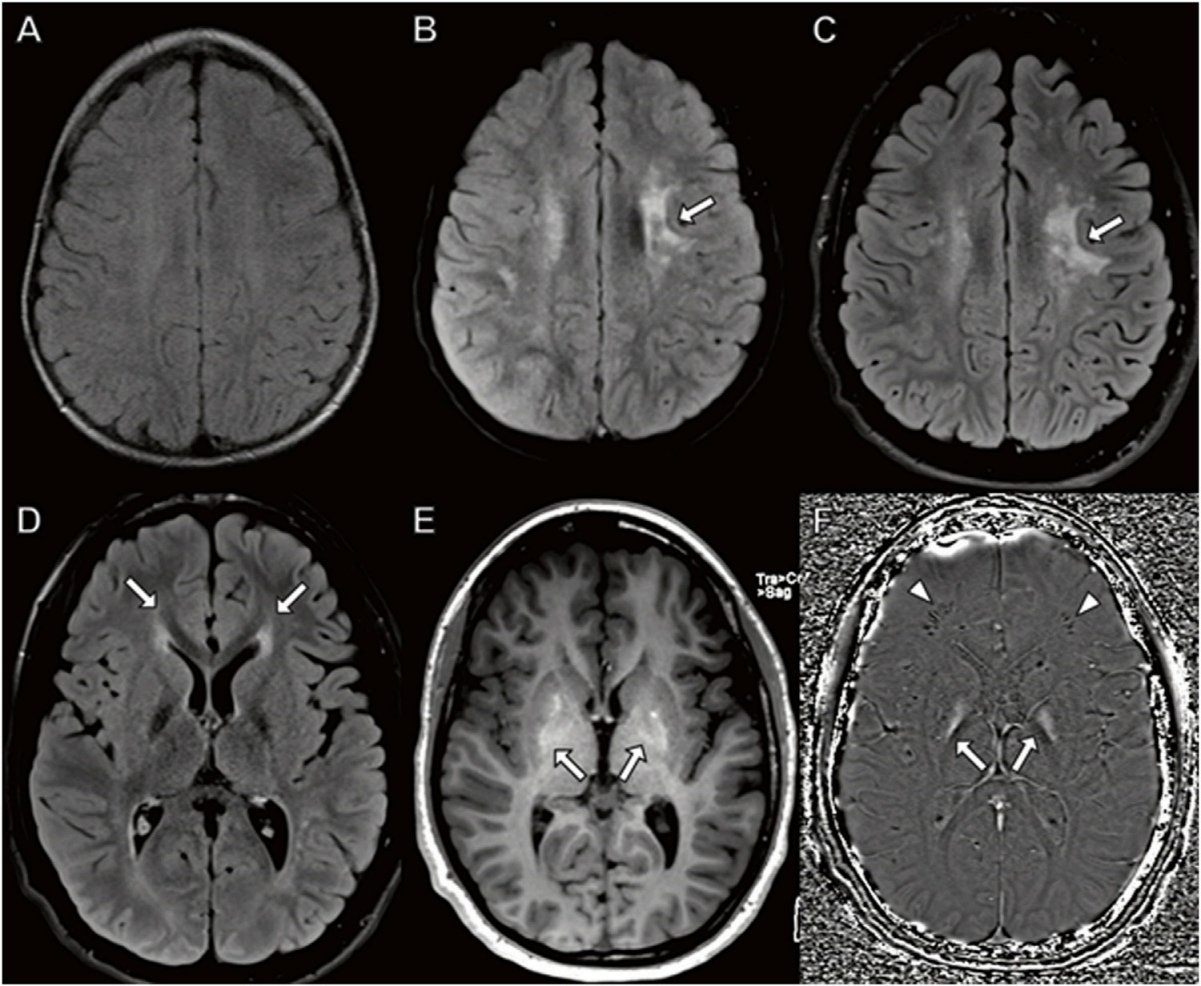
Brain MRI in a boy with *CYP2U1* mutation. (A–C) Axial fluid-attenuated inversion recovery images at (A) 3 years (B) 9 years, and (C) 15 years demonstrate worsening, patchy hyperintense signal in the centrum semiovale involving subcortical U fibers ((B,C) arrows). (D–F) MRI at 15 years with (D) abnormal hyperintense signal extending from the frontal horns of the lateral ventricles reminiscent of the “ear of the lynx” sign (arrows). (E) Abnormal signal hyperintensity in the globus pallidus on axial T1-weighted images corresponding to (F) hyperintense signal on axial susceptibility-weighted phase image (arrows) indicating mineralization. (F) Linear hypointense signal in the frontal white matter representing calcifications in a perivenular distribution (arrowheads).
